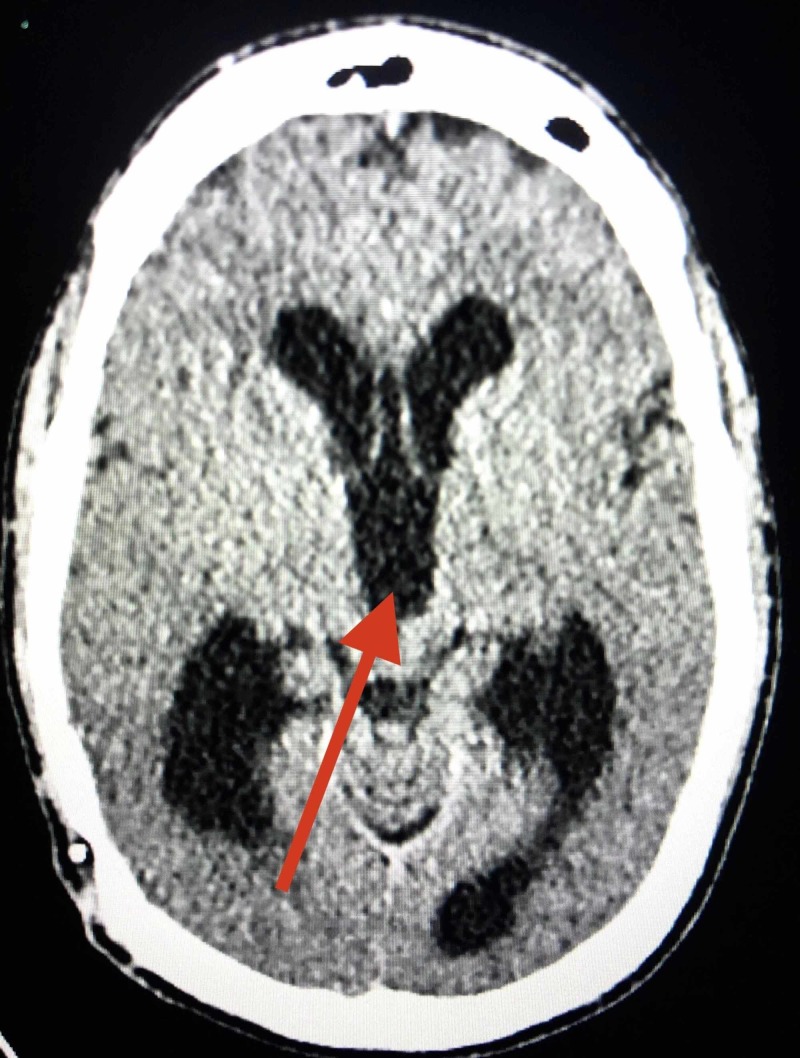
Pre-operative axial computed tomography of the head; arrow indicating dilation of third ventricle
